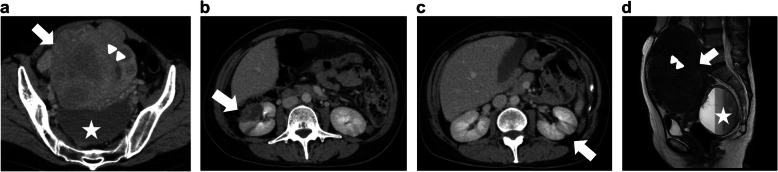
Pelvic imaging on the day of initial bleeding a, d Pelvic contrast-enhanced computed tomography (CT) and magnetic resonance images show adenomyosis (arrowheads), multiple uterine myomas (arrows), and a left ovarian tumor (stars). b-c Pelvic contrast-enhanced CT shows old and new bilateral renal infarctions (arrows)
